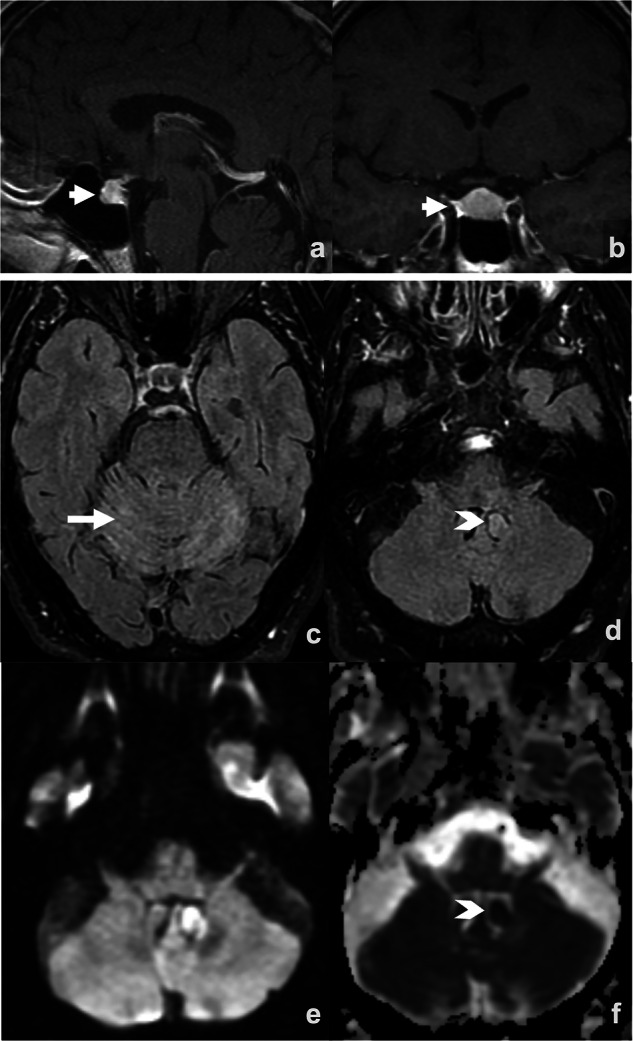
Intracranial immune-related adverse events. Ipilimumab-induced hypophysitis in a patient with brain metastases from melanoma, without concomitant radiotherapy (a, b). Two months after starting immunotherapy, the patient developed panhypopituitarism. An MRI revealed an enlarged, enhancing pituitary gland (arrow in a and b). Pembrolizumab-induced cerebellitis in a patient with brain metastases from melanoma without concomitant radiotherapy (c–f). Five days after treatment began, the patient developed ataxia and dysmetria. An MRI showed T2/FLAIR and diffusion hyperintensity in the cerebellar parenchyma (arrow in c) and left tonsil (arrowhead in d); ADC maps showed cytotoxic edema (arrowhead in f). CSF analysis was positive for anti-GluK2 antibodies
